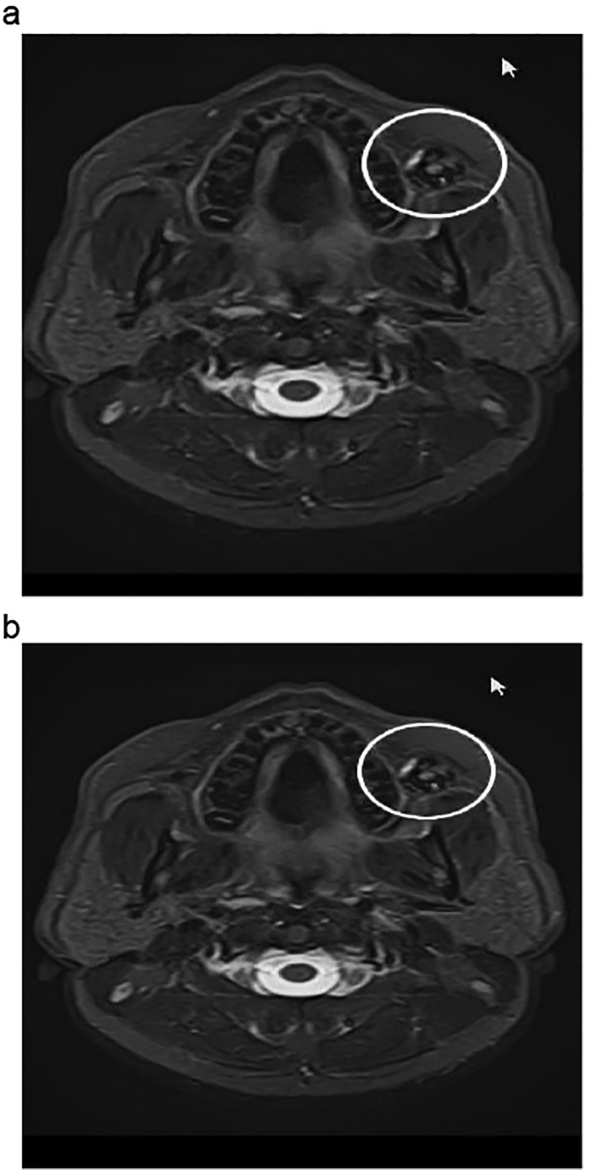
a: MRI of the patient showing enhancing nodular lesion arising from left buccal mucosa. b: MRI of the patient showing enhancing nodular lesion arising from left buccal mucosa.
